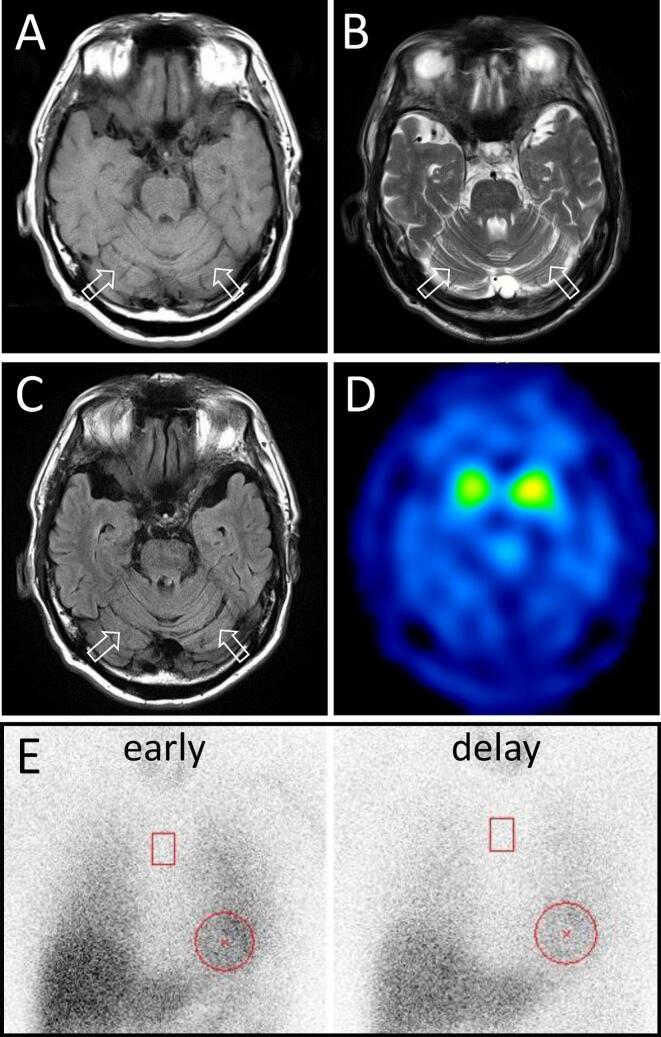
MRI, MIBG-myocardial scintigraphy and FP-CIT SPECT imaging. MRI images. A) T1-weighted, B) T2-weighted, C) FLIAR. MRI showed slight cerebellar ataxia (arrow). D) FP-CIT SPECT imaging revealed symmetrical reduced uptake (Specific Binding ratio: right 2.34, left 2.53). E) Cardiac uptake of MIBG was not reduced (heart/mediastinum ratio: early 3.22, delay 3.22).
